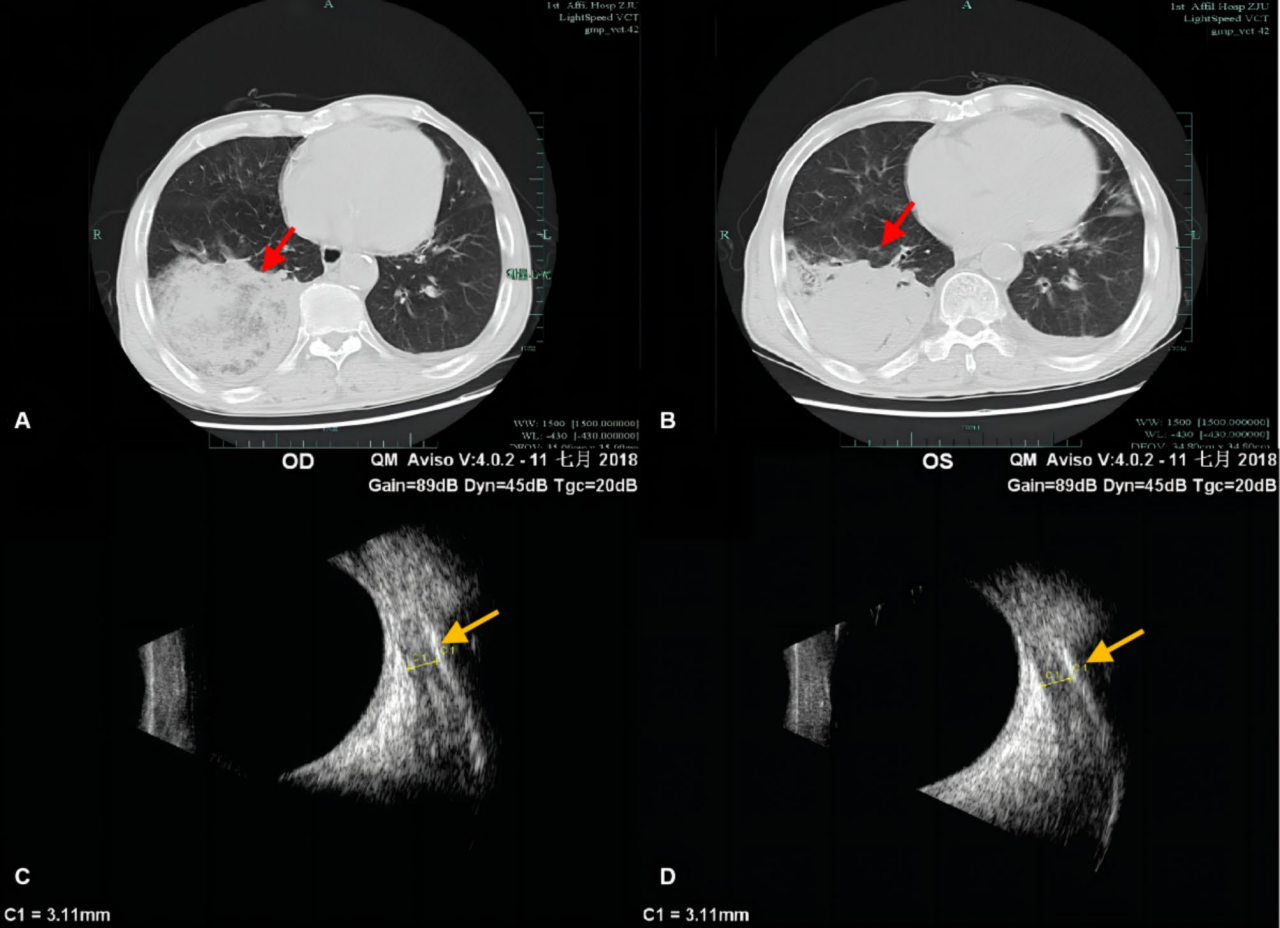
Lung CT and ocular ultrasound of case 3 (A) showing scattered infectious lesions in both lungs and a large patchy consolidation in the right lower lung when the fever occurred (red arrow). (B) Decreased lung inflammation and less extensive consolidation in the right lower lung 1 week later (red arrow). (C, D) Bilateral enlarged extraocular muscles due to TAO (yellow arrow)
